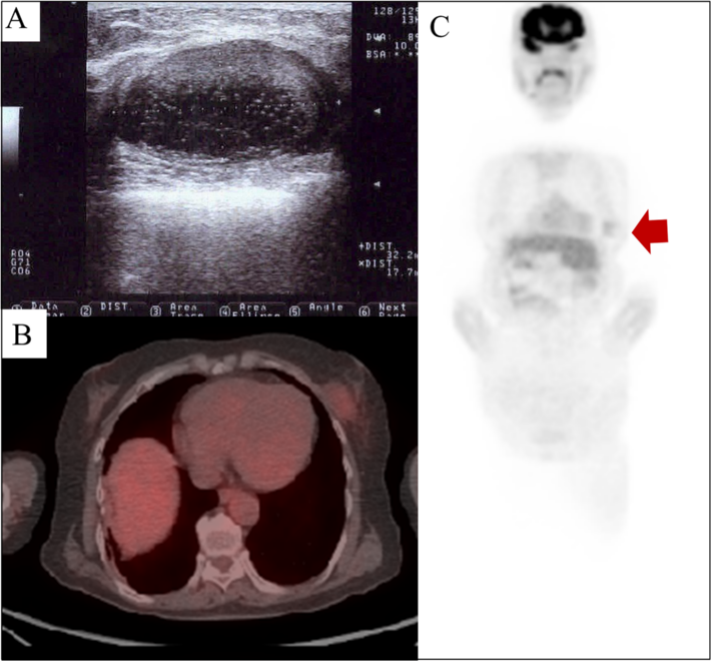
Radiological images of metastatic breast tumor. (A) Ultrasonography demonstrated a 3.2 × 2.8 cm irregular, hypoechoic, heterogeneous mass in the breast. Macrocalcifications were not observed. (B) Axial PET/CT fusion images revealed the accumulation to be comparatively high (SUV of 2.1) in the tumor within the left breast. (C) With the exception of the breast, accumulation suggestive of metastasis was not found on PET/CT.
